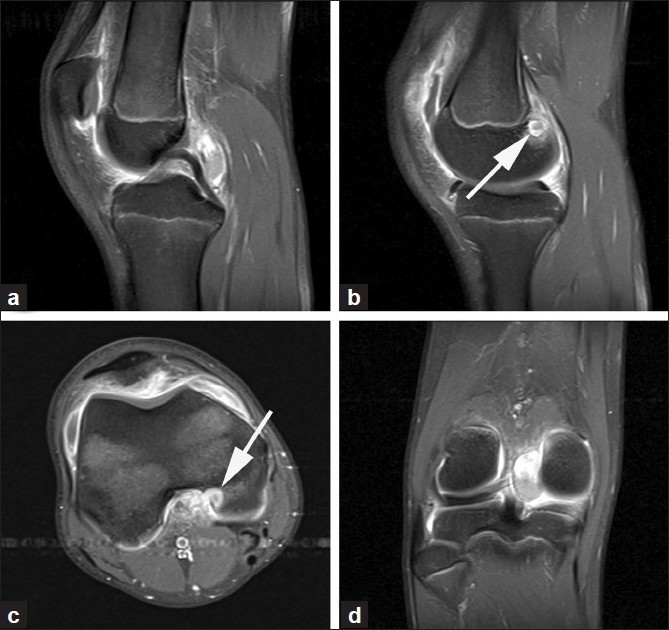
MRI sagittal T1W images showing the lesion is posterior to the posterior cruciate ligament (a). Another view (b) indicates the lesion (arrow) eroding into the femoral condyle. Axial image (c) shows the lesion (arrow) eroding into the posterior medial femoral condyle. Coronal view (d) shows the lesion as it lies laterally to the medial femoral condyle.
